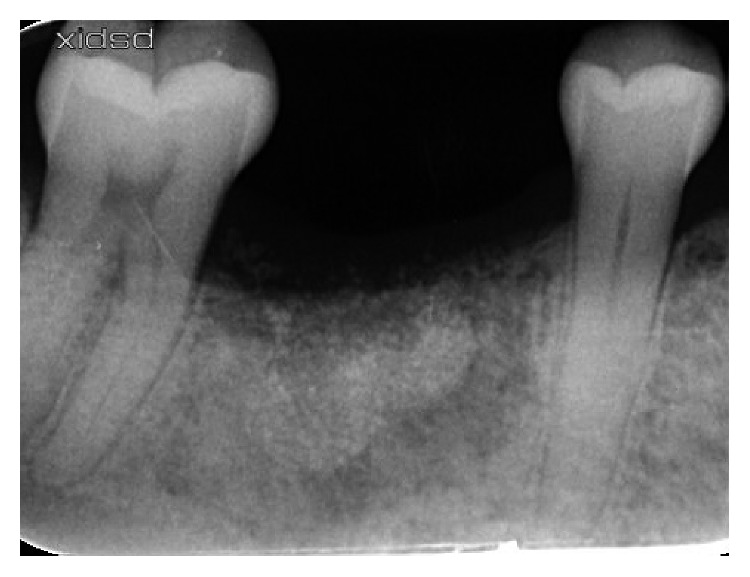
Periapical X-ray at 12 weeks showing that the site is occupied by newly formed hard tissue.
